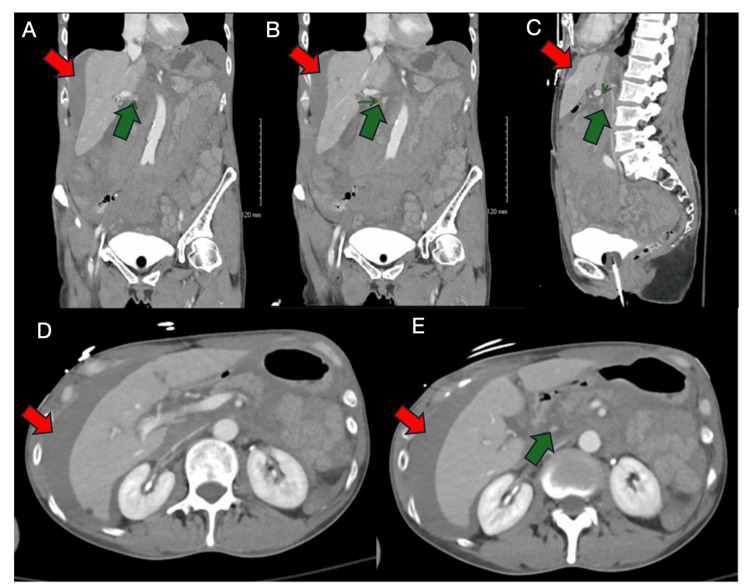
Initial multiplanar CT imaging on day 0 (axial, coronal, and sagittal views). CT images demonstrate a large mesenteric mass suspicious for hematoma, with differential considerations including lymphadenopathy or lymphoma (A) Coronal view (anterior): moderate hemoperitoneum (red arrow) with a small focus of contrast extravasation (green arrow) in RUQ. (B) Coronal view (posterior): moderate hemoperitoneum (red arrow) with a small focus of contrast extravasation (green arrow) in RUQ. (C) Sagittal view: hemoperitoneum (red arrow) with multiple foci of contrast extravasation (green arrow) in RUQ. (D) Axial view (superior): moderate hemoperitoneum (red arrow) without a definite extravasation focus. (E) Axial view (inferior): hemoperitoneum with a small focus of contrast extravasation in RUQ. CT: computed tomography; RUQ: right upper quadrant
